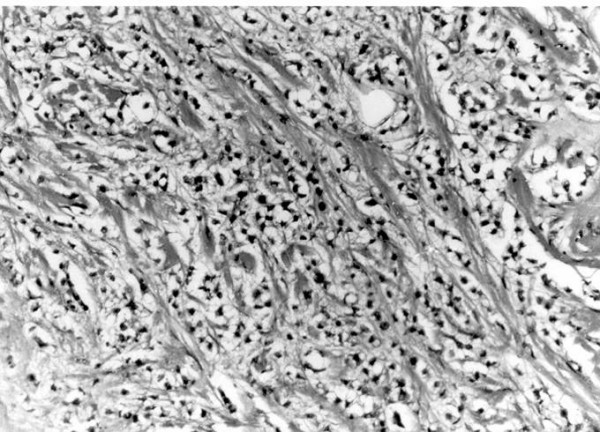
Five years later (1999) she presented with back pain. Radiographs of the spine revealed sclerosis in the body of the fifth lumbar vertebra. It was thought that this was possibly metastatic disease but a bone scan was reported as normal. Mammograms done every year on the remaining breast were also described as normal. A year later (2000), about 6 years post-mastectomy, there was no evidence of recurrence but she was continued on Tamoxifen. In February 2001, seven years after her initial presentation she presented with pain in the volar aspect of her right wrist for 2–3 months with restriction of movement. She was seen by the orthopaedic surgeons who found mild swelling with pain on radial deviation but no pain on compression, no anteroposterior laxity and no wasting. Radiography of the wrist showed a lucent area in distal ulna. This was confirmed by nuclear bone scan showing a "hot area" corresponding to the area of lucency. Again this was thought to be metastatic given the increase in radioisotope uptake which is not usual for the lytic bone disease in myeloma. Computerised Tomography scan showed a lesion extending into ulnar styloid process but no cortical destruction. Haematological and blood biochemical tests revealed a haemoglobin of 10 g/dl, white blood cell count of 4.0 × 10^9^/l, platelet count of 164 × 10^9^/l. Her serum globulins were elevated at 68 g/l, calcium was not elevated and the erythrocyte sedimentation rate was elevated at 52 mm/hr. Serum Protein electrophoresis revealed a monoclonal band in γ region with decreased normal immunoglobulins. Bone marrow examination revealed 60 % plasma cells (figure 2). Multiple Myeloma was diagnosed based on the presence of >30% plasma cells in the bone marrow, the monoclonal band and the lytic skeletal lesion. She was treated with melphalan, prednisone and allopurinol which she tolerated well and the pain in the wrist improved. She was advised then by the haematologists to discontinue tamoxifen as she had already had 5 years of treatment.
